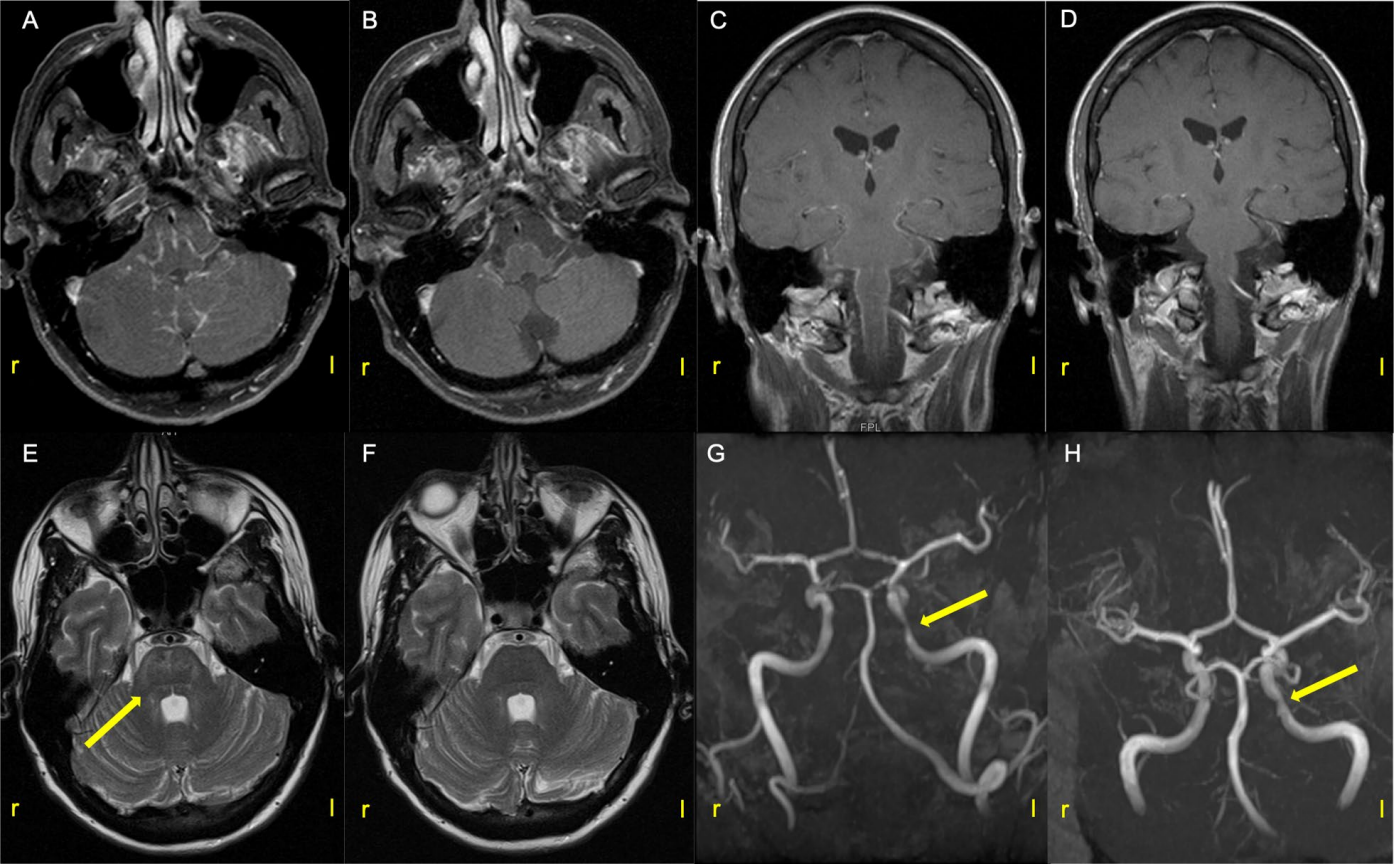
MRI of the brain shows gadolinium-enhancement along the brainstem, cerebellum, occipital and temporal sulci (A and C before treatment), which decreased during the course (B and D ten days after). T2-hyperintesities could be detected in the brainstem up to the diencephalon (primarily thalamus and parts of the capsula interna on both sides). E shows the MRI before start of treatment, F four months after. G shows MR-TOF angiography with distal ACI stenosis on the left, H 4 months later (arrows). A, B axial T1; C, D coronal T1; E, F axial T2; G, H MR-TOF angiography
